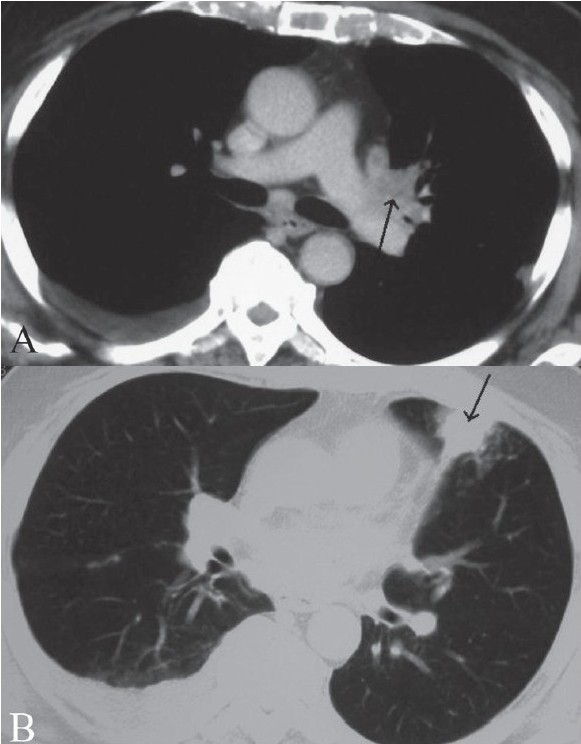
Soft tissue (A) and lung window (B), contrast-enhanced CT images of the chest show necrotic lymphnodes in the left hilar region (arrow) with consolidation in the left lung (arrow)
